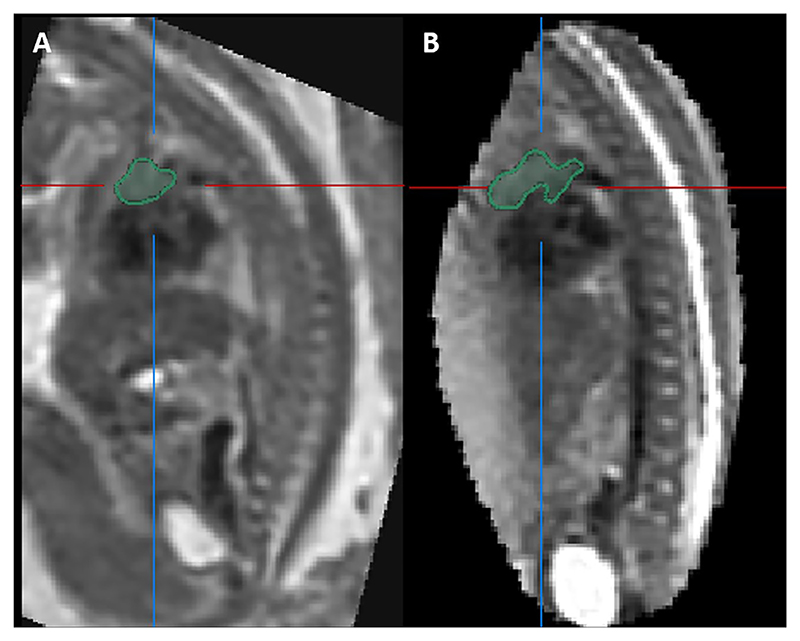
Slice-to-volume reconstructed MRI images of the fetal thorax from two fetuses showing the segmented fetal thymus gland in the sagittal plane. Scan A conducted at 24 + 6 weeks gestation. Scan B conducted at 24 + 2 weeks gestation.
